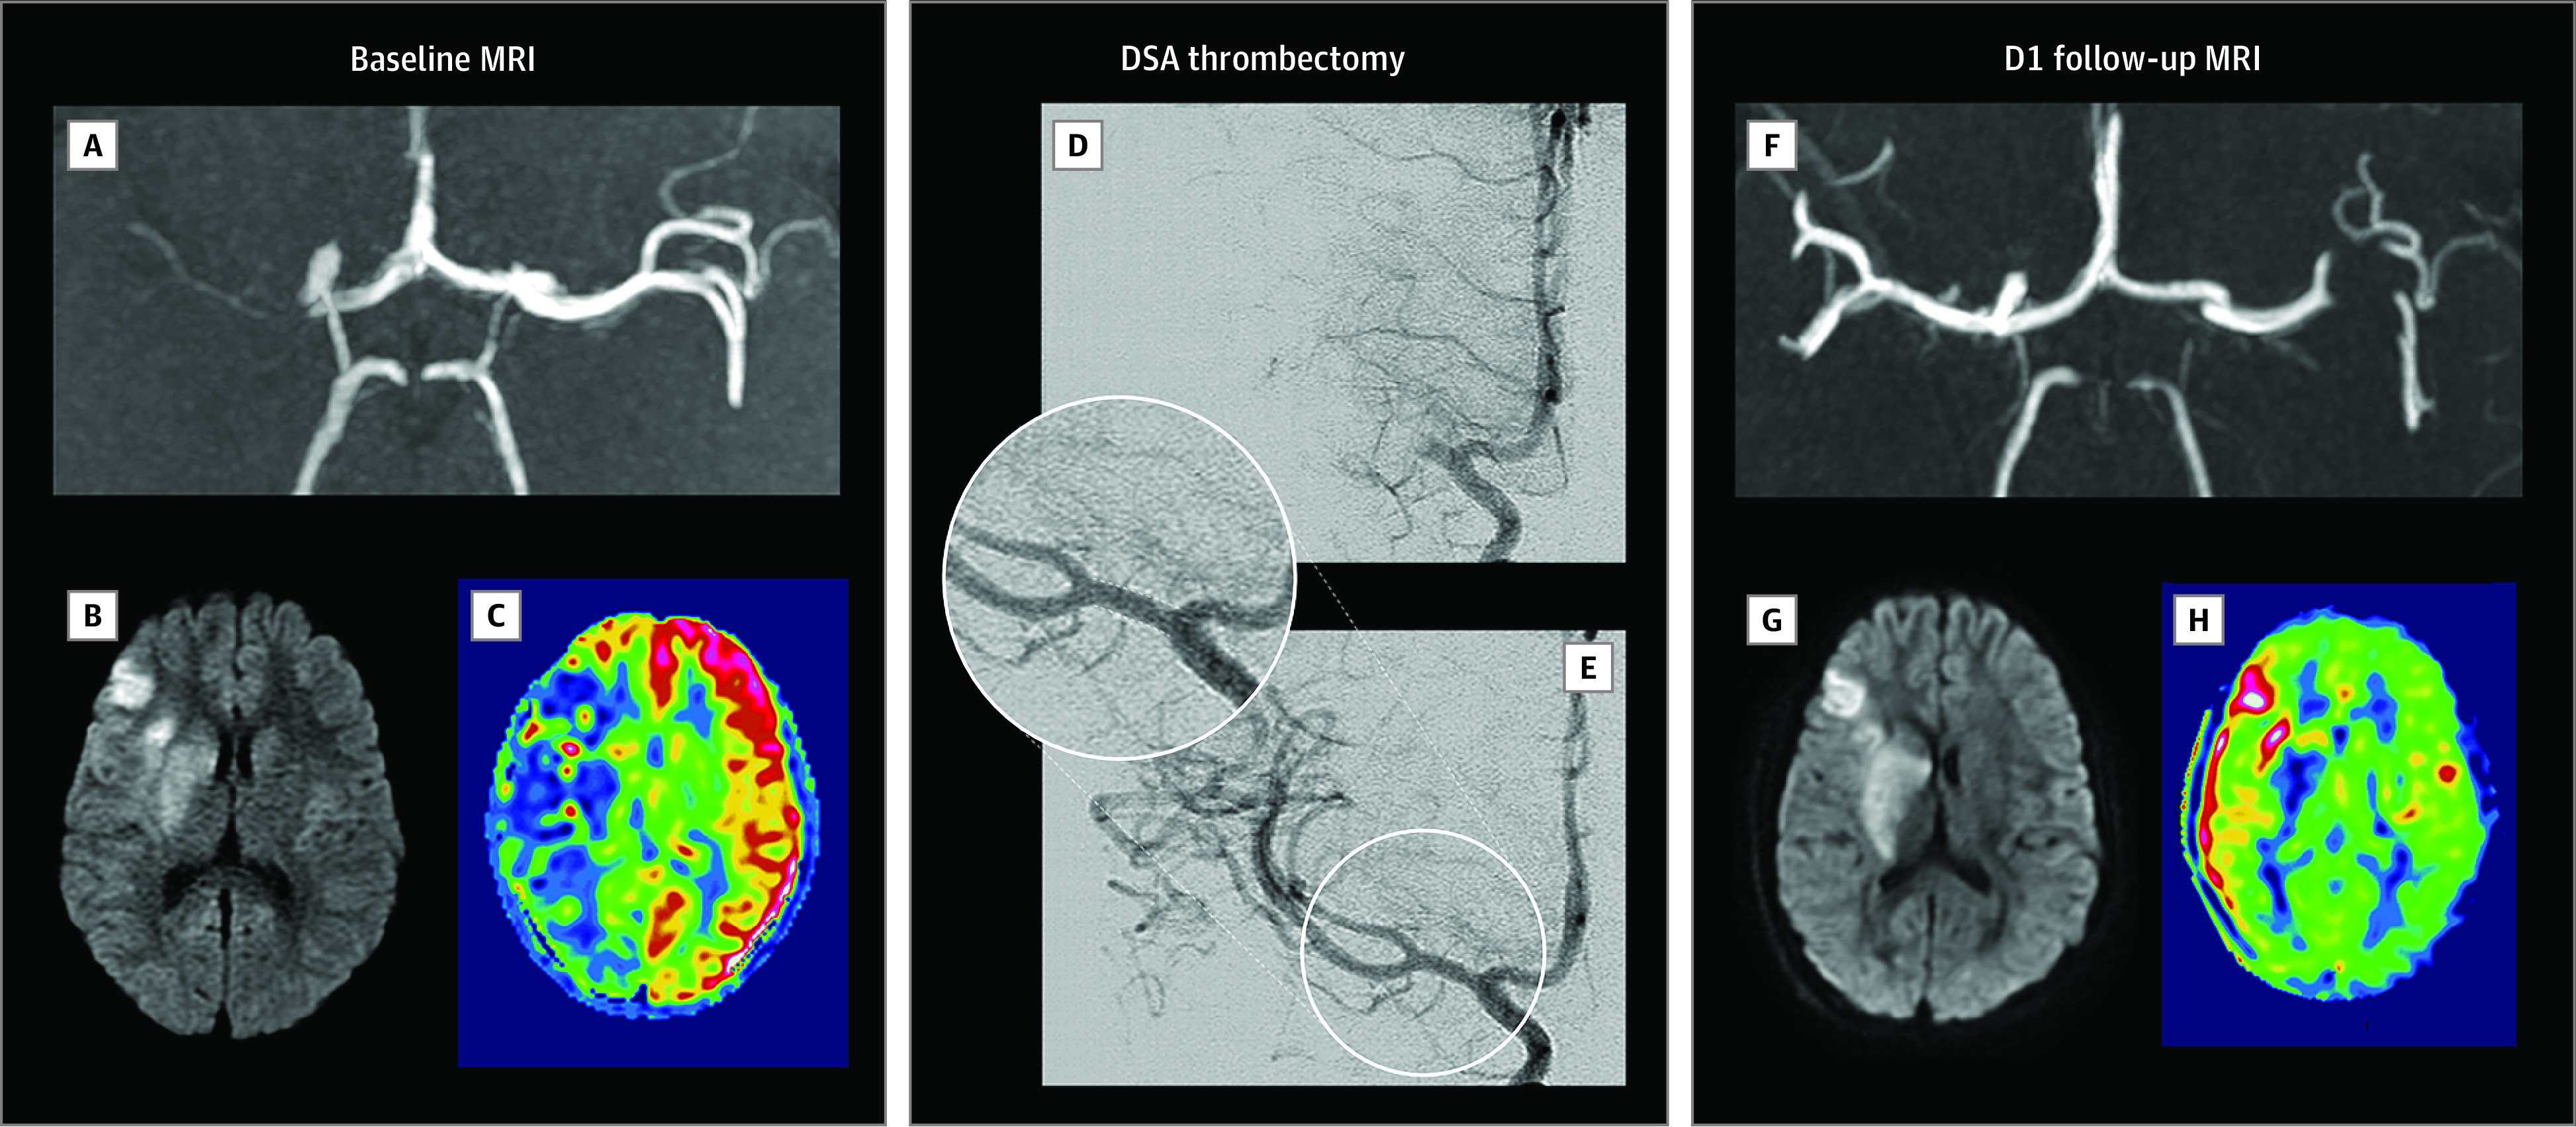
Pediatric Acute Ischemic Stroke of Cardioembolic Origin Treated With Mechanical Thrombectomy Acute ischemic stroke in a child aged 8 years with a clinical history of embolic heart disease presenting with sudden left hemiplegia and dysarthria. A-C: Baseline magnetic resonance imaging (MRI) performed 5 hours and 20 minutes after stroke onset. A, Axial maximum intensity projection reconstruction of time-of-flight angiography showing the occlusion of the proximal M1 segment of the right middle cerebral artery (MCA). B, Axial diffusion image showing hyperintensity limited to right caudate and lenticular nuclei, insula, and frontal cortex. C, Axial arterial-spin-labeling with cerebral blood flow cartography (ASL-CBF) showing markedly decreased perfusion throughout the right sylvian territory compared with the left side. D-E: Periprocedural digital subtraction angiography (DSA) images. D, Initial anteroposterior images after opacification of the right carotid territory confirming the occlusion (proximal M1 segment) of the right MCA. E, Final anteroposterior images after mechanical thrombectomy with stent retriever showing the patency without stenosis of the right MCA. F-H: Follow-up MRI performed 24 hours (D1) after the mechanical thrombectomy. F, Axial maximum intensity projection reconstruction of time-of-flight angiography showing restored patency of the right MCA territory. G, Axial diffusion image with no major extension of the ischemic core. H, Axial ASL-CBF cartography showing restored perfusion in the right MCA territory.
